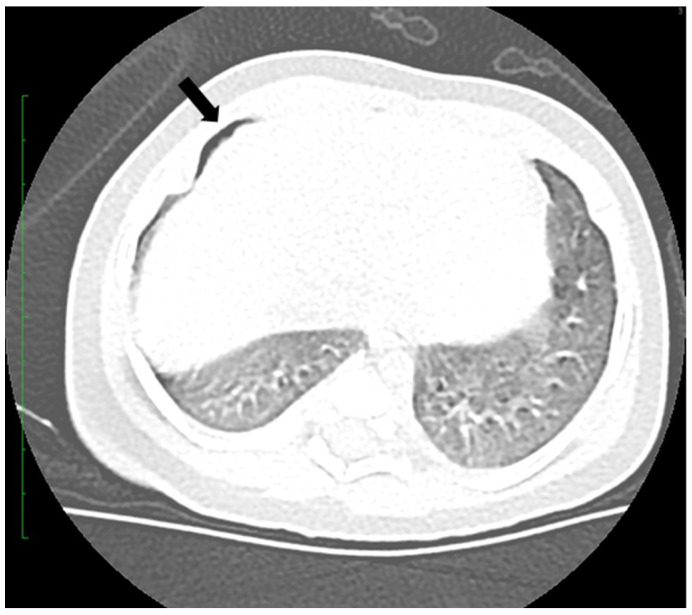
Axial CT scan showing with arrow small right pneumothorax.
